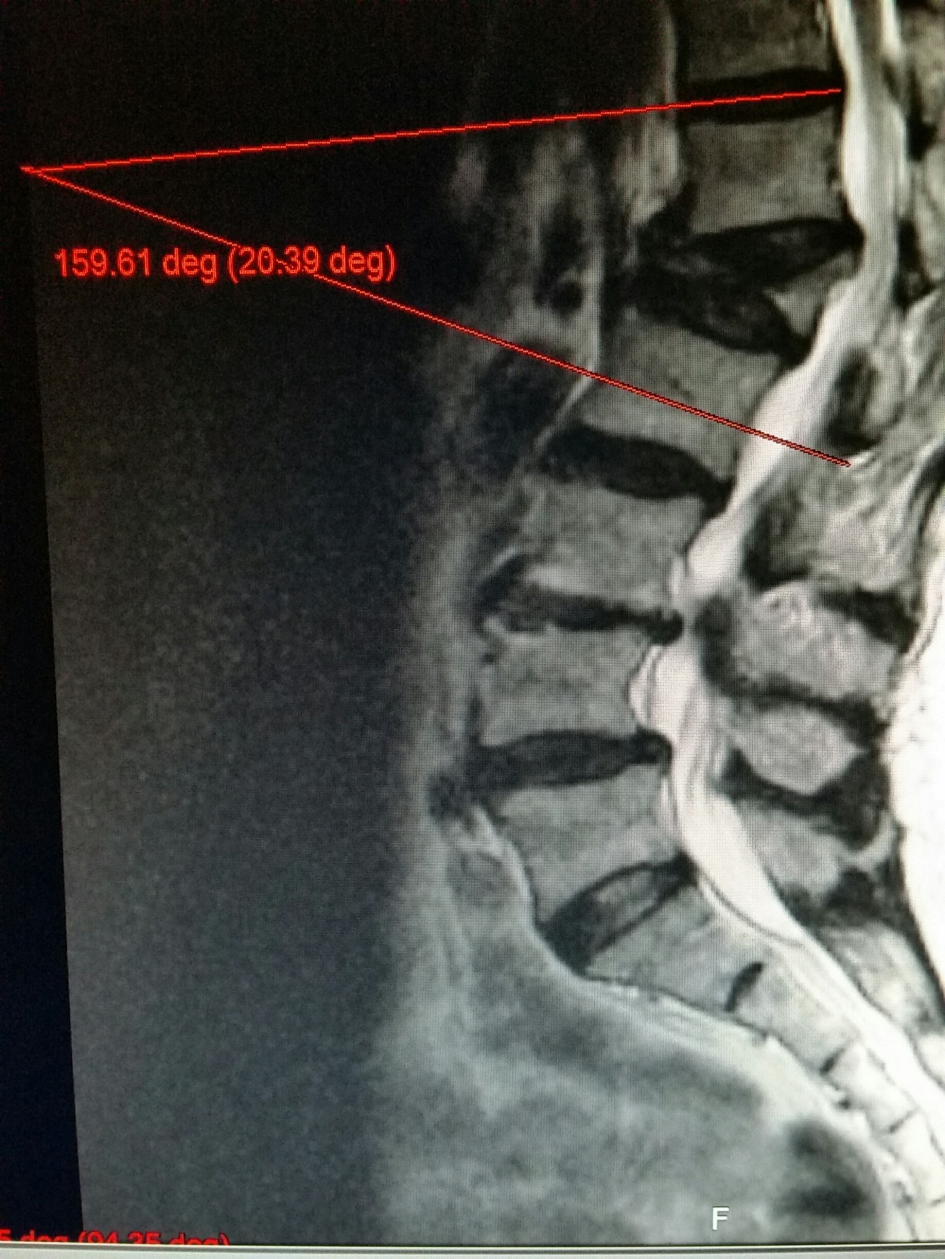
MRI sagittal view demonstrating kyphotic angulation after progressive collapse of an untreated vertebral compression fracture
